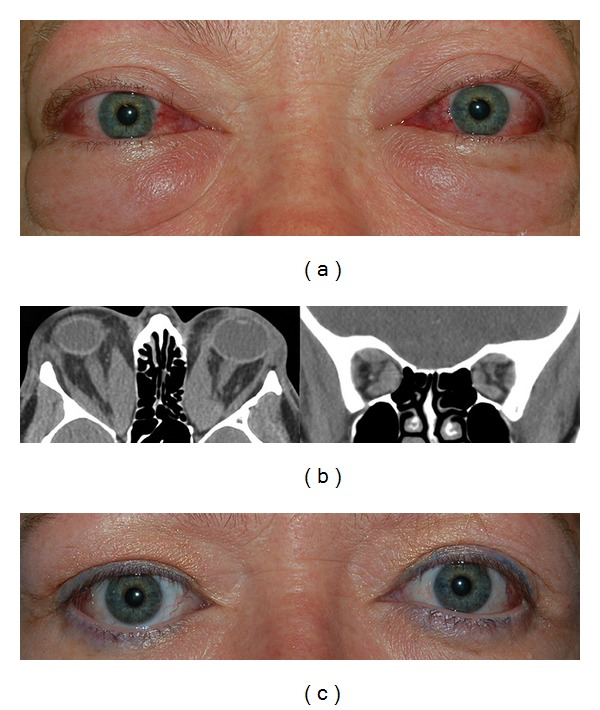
(a) A 49-year-old female who failed intravenous and oral corticosteroids with bilateral optic neuropathy and CAS 9 in the right eye and 10 in the left eye. (b) Computed tomography of her orbits showed enlarged extraocular muscles and orbital apex crowding. (c) 12 weeks after bilateral orbital decompression with resolution of optic neuropathy and CAS 2 in both eyes.
